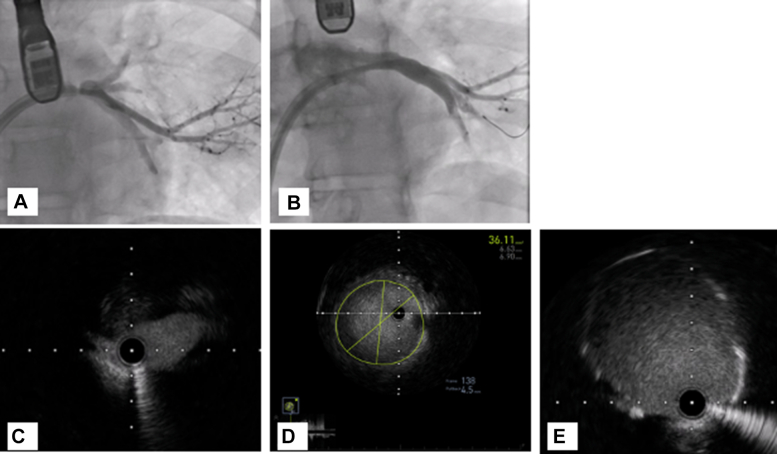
Angiography and IVUS Before and After Stenting (A) Selective LIPV angiography before stenting. (B) Selective LIPV angiography after stenting. (C) IVUS showing the lesion at the proximal LIPV with no calcifications. (D) Distal reference on IVUS. (E) Poststenting IVUS at the lesion site with well-expanded and well-apposed stent. IVUS = intravascular ultrasound; LIPV = left inferior pulmonary vein.
